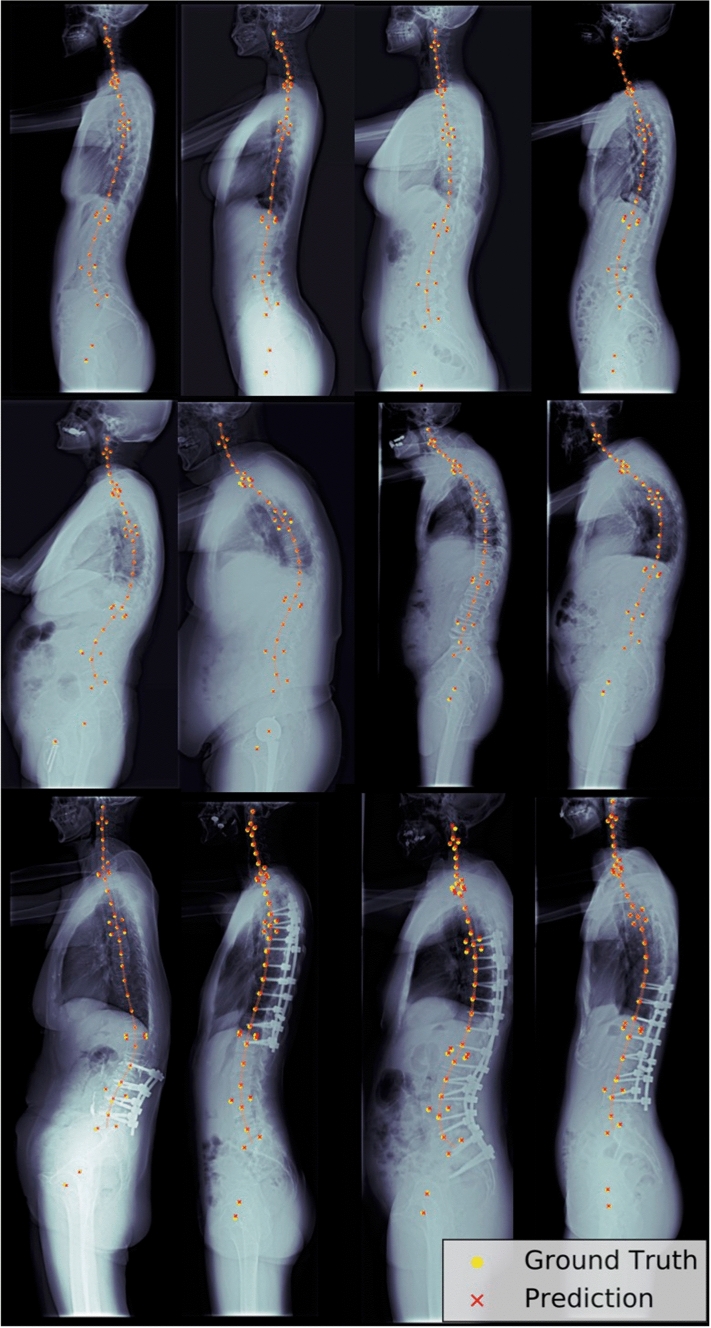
Prediction examples. Examples of model predictions in images with different spinal pathologies, i.e. scoliosis, kyphosis, or implant. For each radiograph, the predicted locations (red crosses) and human-annotated locations (yellow circles) were plotted. We also approximated the spinal curves (orange line) by interpolating the predicted locations of vertebral centres.
